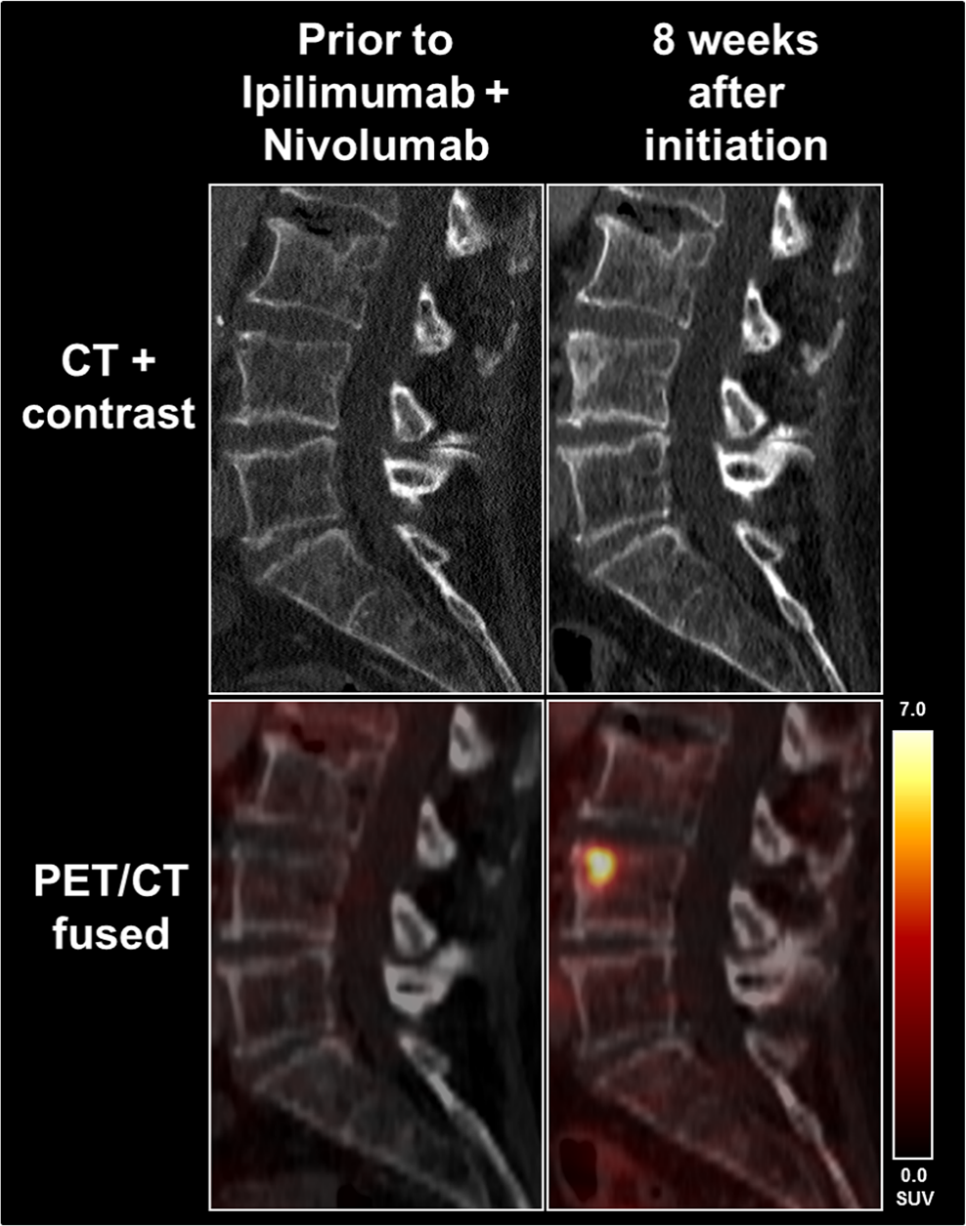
A 77-year-old female patient showed a new osteoblastic lesion on follow-up CT during therapy with Ipilimumab and Nivolumab. According to RECIST 1.1, this is not rated as PD. However, a high PSMA expression could be seen on PET indicating this lesion to be a vital metastasis rather than an avital osteoblastic reaction to therapy. Consequently, this was rated PD_PET_, although the other tumoral lesions showed stable uptake on PET
